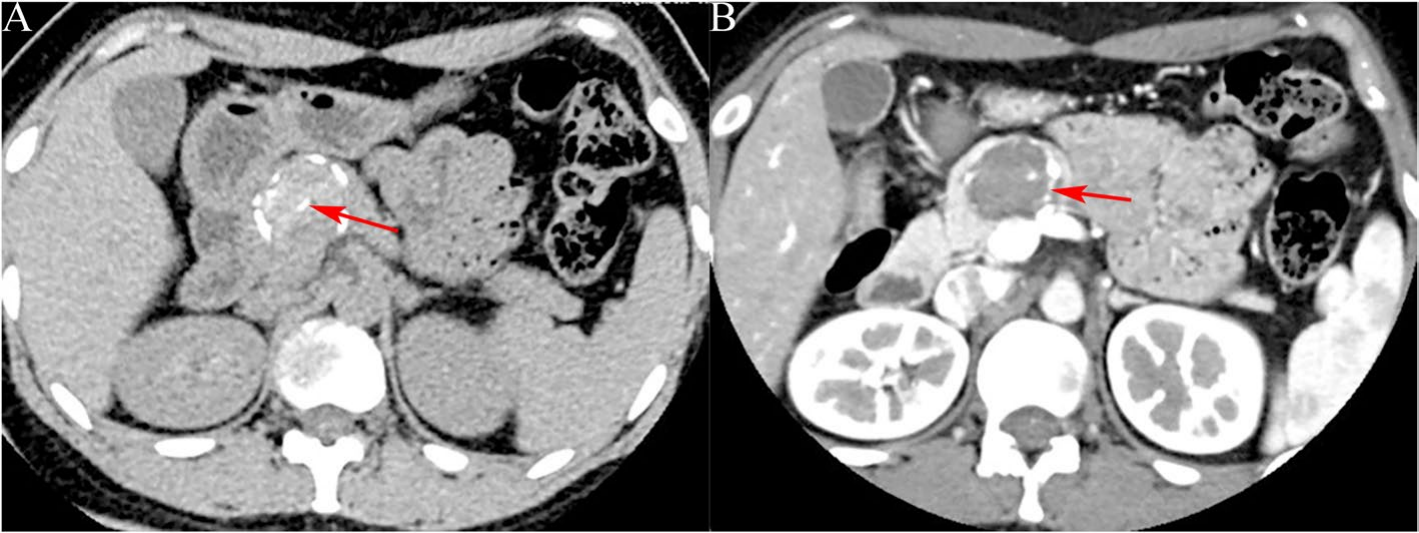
A 47-year-old female with a solid pseudopapillary tumor. a Plain CT scanning shows that the SPTP is located on the pancreatic head, with isodensity and central punctate calcifications (long arrow) and marginal semiarc calcifications of the tumor; b CT target scanning shows that the solid components in the tumor center are mildly enhanced (long arrow), to a lower degree than the surrounding normal pancreatic parenchyma during the pancreatic parenchymal phase
